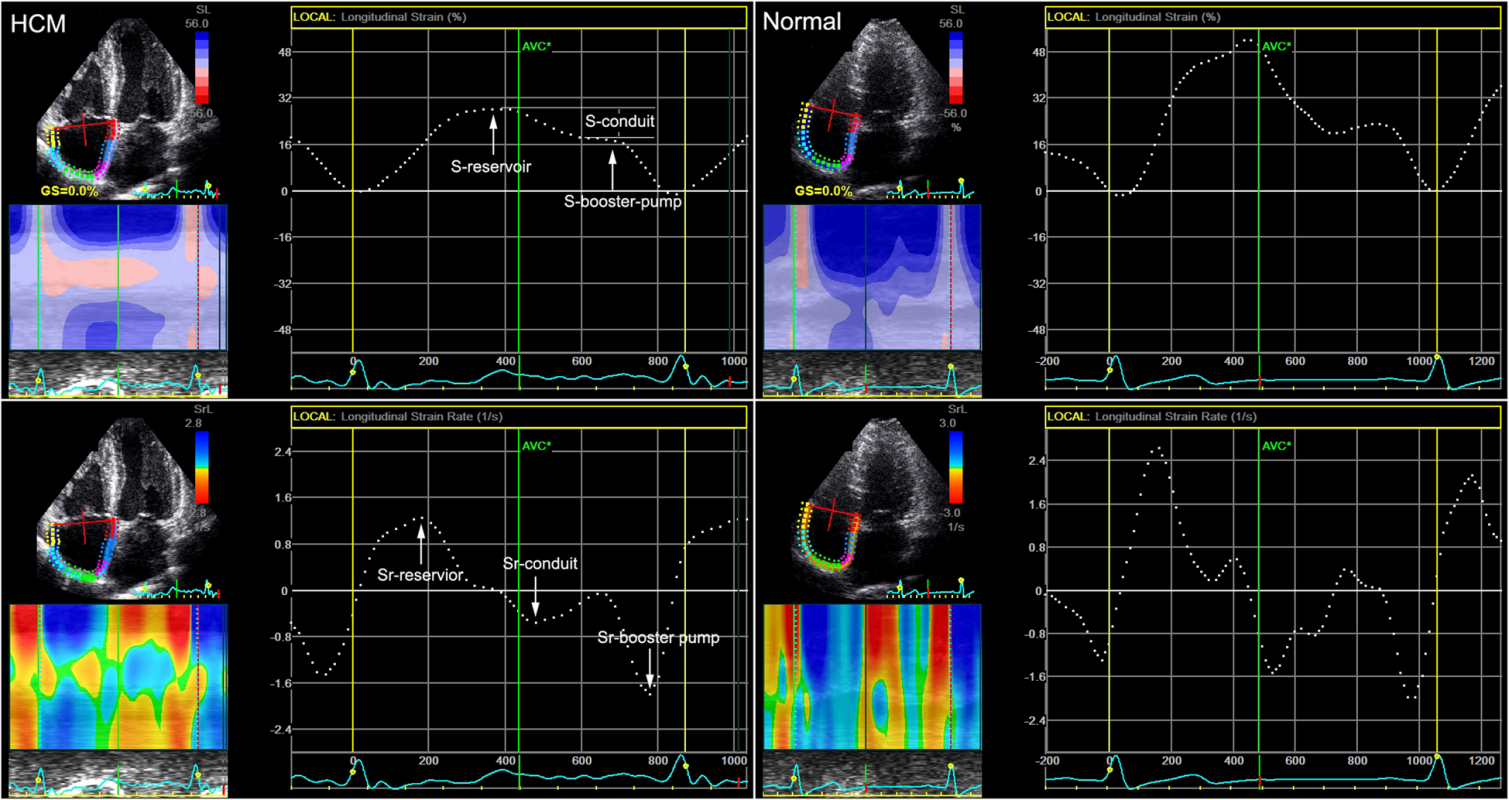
RA functions in apical 4-chamber views in HCM patients and normal controls by using EchoPAC software. S-reservoir, S-conduit and S-booster pump, as well as Sr-reservoir, Sr-conduit and Sr-booster pump, corresponded to the reservoir, conduit and booster pump functions of RA, respectively
